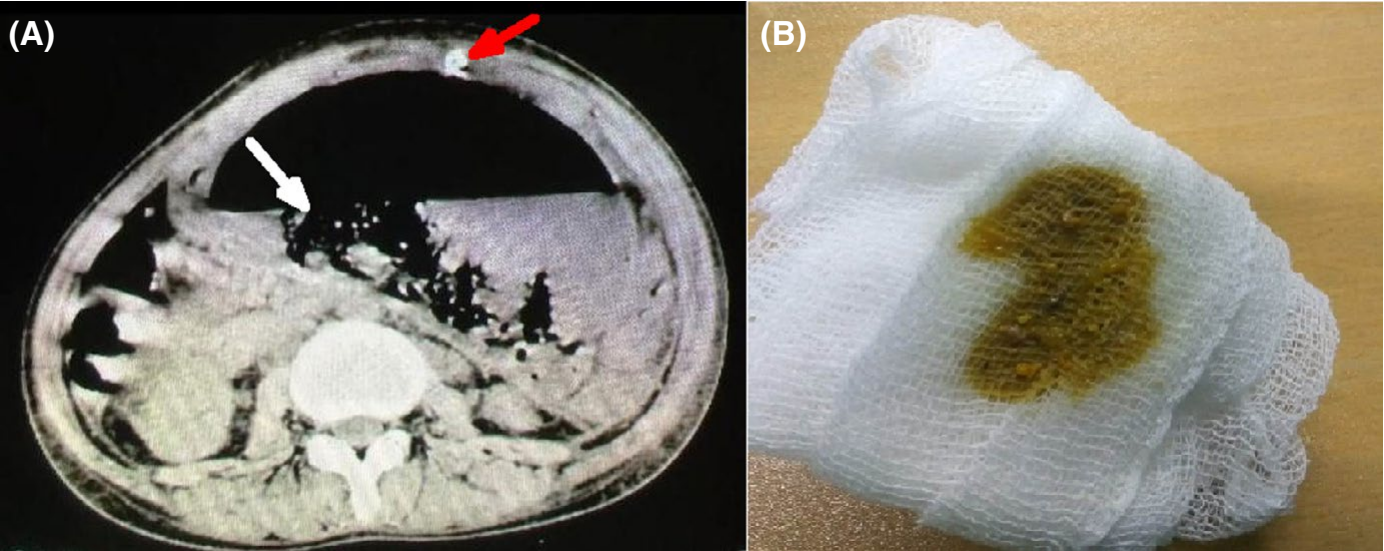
A, Computed tomography (CT) abdomen showing massive air in the pancreatic necrosis (*white arrow*). Note the pigtail catheter tube in the abdominal wall (*red arrow*). B, The feculent content drained by the pigtail catheter suggesting colonic fistula
